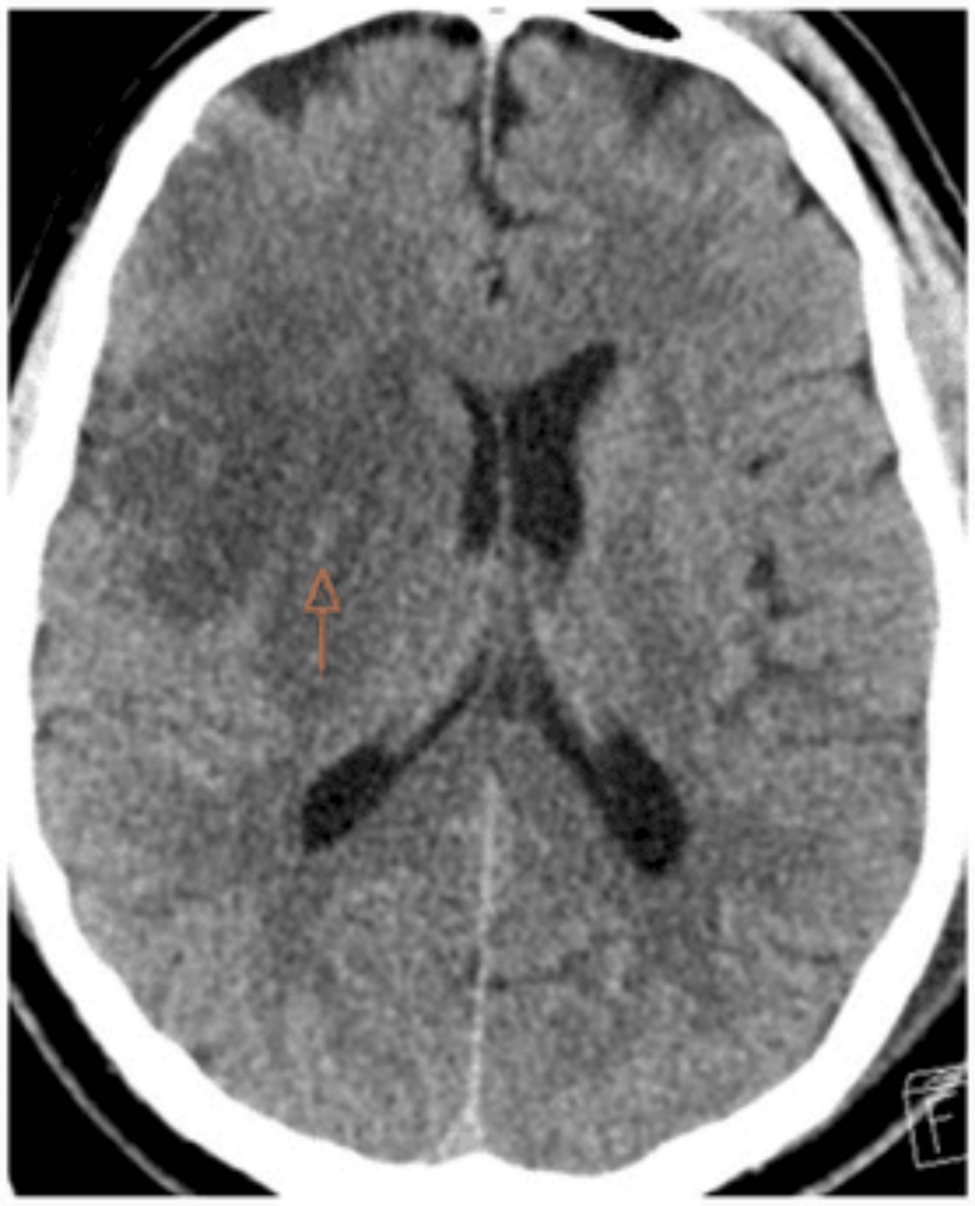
CT of the head without contrast 24-hour postinterventional treatment demonstrating hemorrhagic infarction type I (orange arrow) CT: computed tomography
